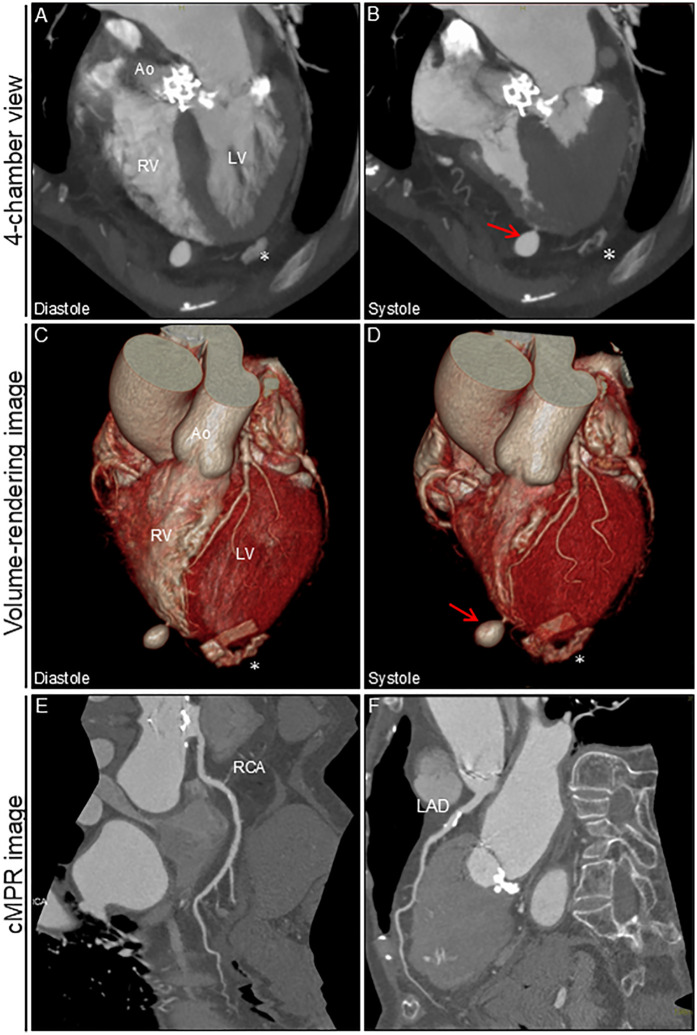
Cardiac computed tomography angiography image after ablation. 4-chamber view and volume-rendering images during diastole (A,C) and systole (B,D). Outpouching with a narrow neck at the apex of the right ventricle is markedly prominent during systole (arrows). No significant pericardial effusion was observed. cMPR images of RCA (E) and LAD (F) * Denotes the remnants of felt in the left ventricular apex after transapical aortic valve replacement. Ao, aorta; cMPR, curved multi-planar reformation; LAD, left anterior descending artery; LV, left ventricle; RCA, right coronary artery; RV, right ventricle.
